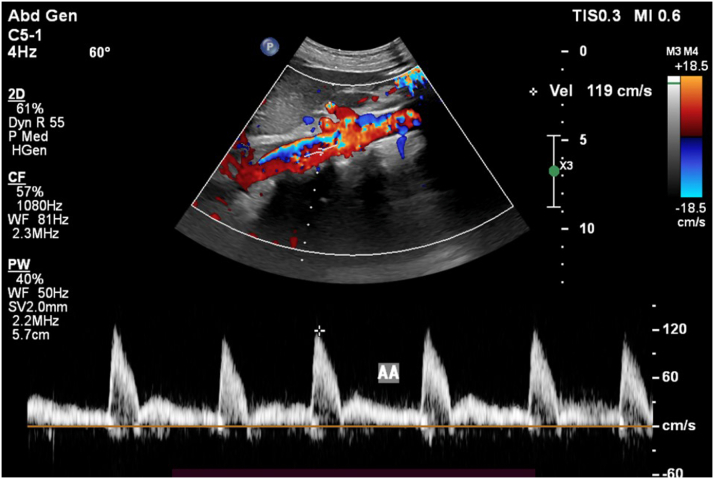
1-Year Follow-Up Post Percutaneous Transluminal Angioplasty Distal Aortic Intervention Through Duplex Abdomen Ultrasound
